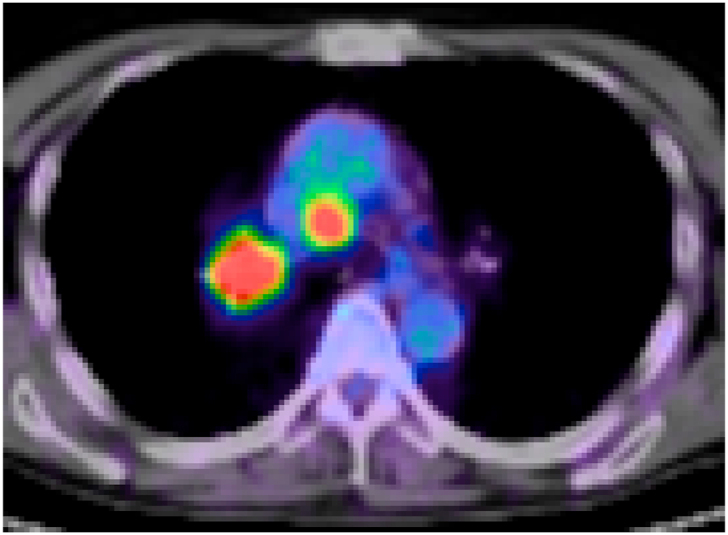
Fused PET/CT image shows FDG concentration in the paratracheal bulky swollen lymph node and primary lung tumor.
